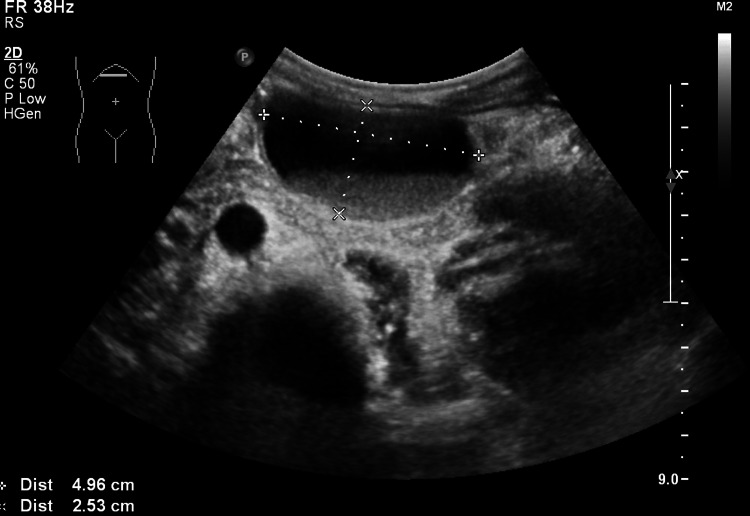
Abdominal ultrasound showing a thin-walled cyst with a sediment layer, measuring approximately 5 × 3 cm, located adjacent to the upper part of the pancreas at the junction of the body and tail.
